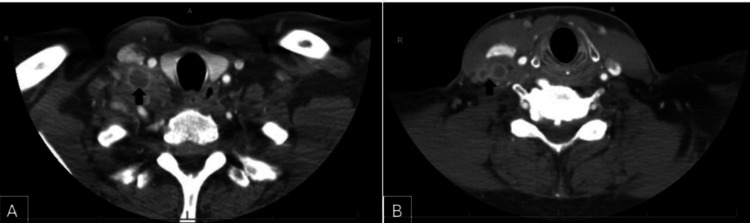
CT findings of the neck mass. CT images (A, B) show multiple necrotic lymph node conglomerates, indicated by black arrows.
